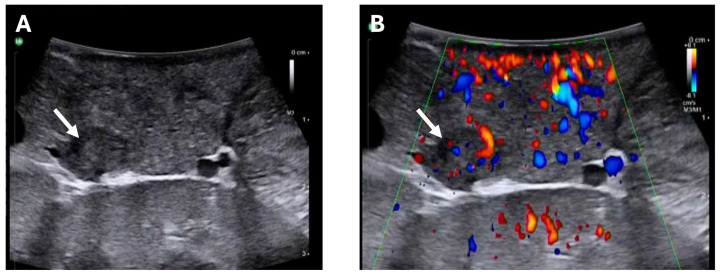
Intraoperative robot-assisted ultrasound of occult insulinoma. (A) B-mode and (B) colour-coded doppler sonography of the same patient with occult insulinoma localising an intrapancreatic, hypervascularised lesion (arrows).
